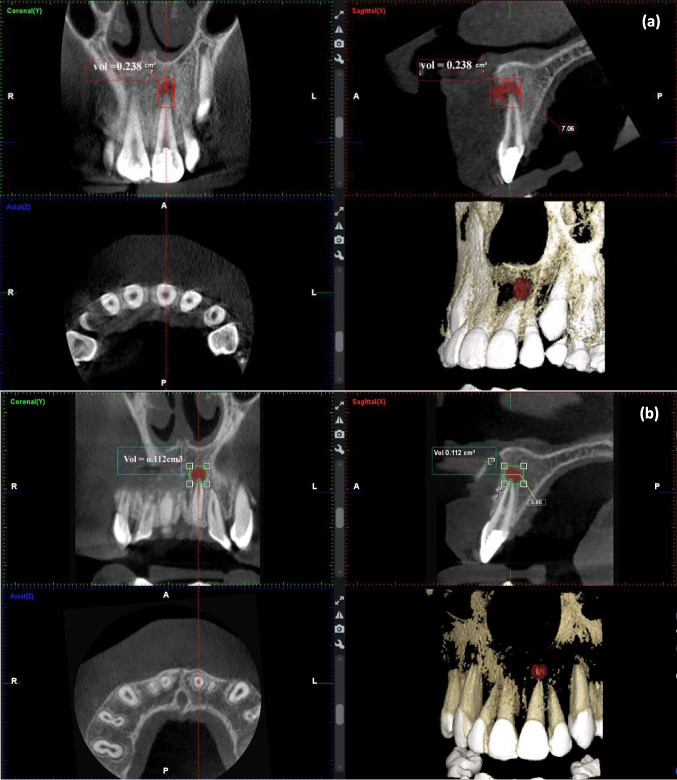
Cone-beam computed tomography (CBCT) scans of periapical lesion diameters (PALD) and periapical lesion volumes (PALV) of the maxillary left central incisor of a 13-year-old female retreated with induced blood clot (BC) at a baseline; the maxillary left central incisor with PALD = 7.06 mm and PALV = 0.238 cm^3^. b Reduction of periapical lesion size with PALD = 3.85 mm and PALV = 0.112 cm^3^ after 12 months of follow-up
